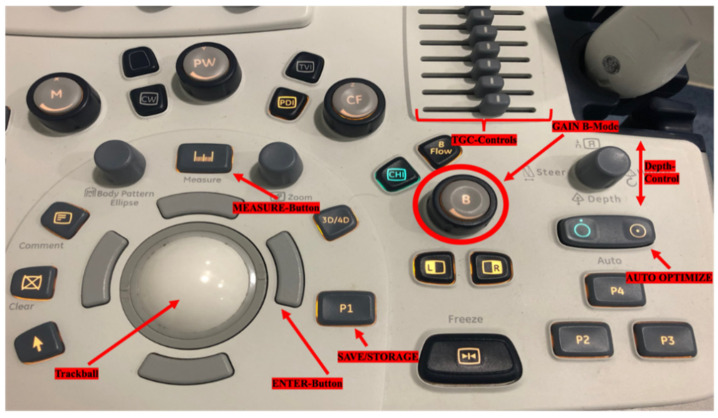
Knobology of a standard ultrasound (US) device. Useful buttons for simplified US usage are marked in red. Time gain controls (TCG) may be all set in the middle position. The depth is varied by the upper and lower lever actions (far right, up). The “Auto Optimize” button harmonizes the image quality. P1 can be programmed to save/store images (in freeze) or cine loops (in a nonfrozen visualization).
